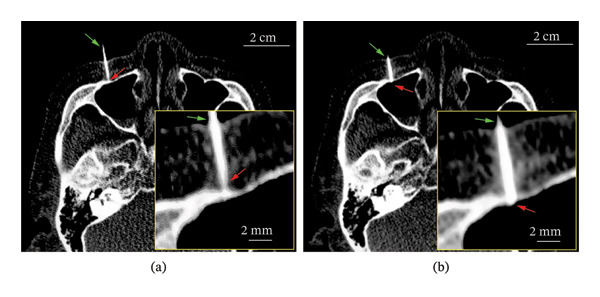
CT imaging of radiofrequency needle placement for infraorbital nerve targeting. (a) Axial CT scan showing the radiofrequency needle (green arrow) approaching the infraorbital foramen (red arrow), with the infraorbital canal and adjacent bony structures clearly visible. (b) Axial CT scan confirming the radiofrequency needle tip (green arrow) successfully inserted into the infraorbital canal. The red arrow shows proper intraforaminal needle placement. Insets display magnified views of the infraorbital foramen region in both panels for enhanced anatomical clarity and visualization of needle trajectory. These images demonstrate accurate needle guidance under CT for safe and effective infraorbital nerve radiofrequency thermocoagulation.
